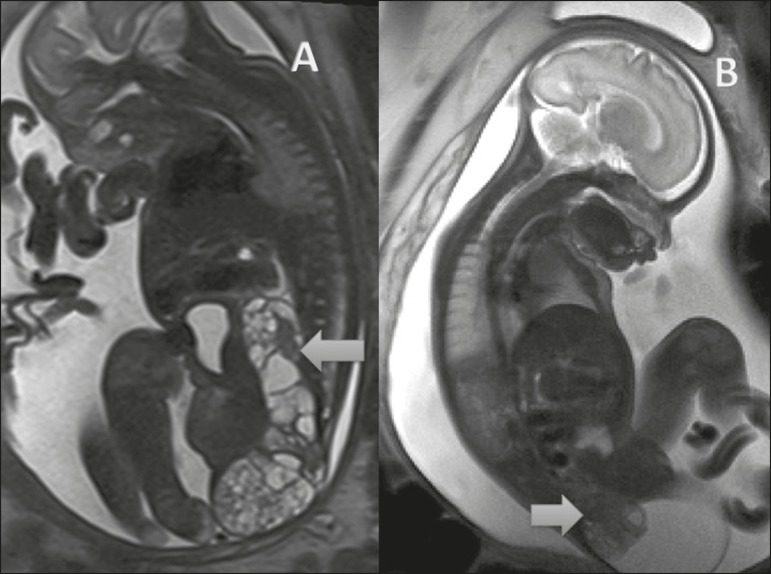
Sacrococcygeal teratoma. A: Sagittal T2-weighted sequence
